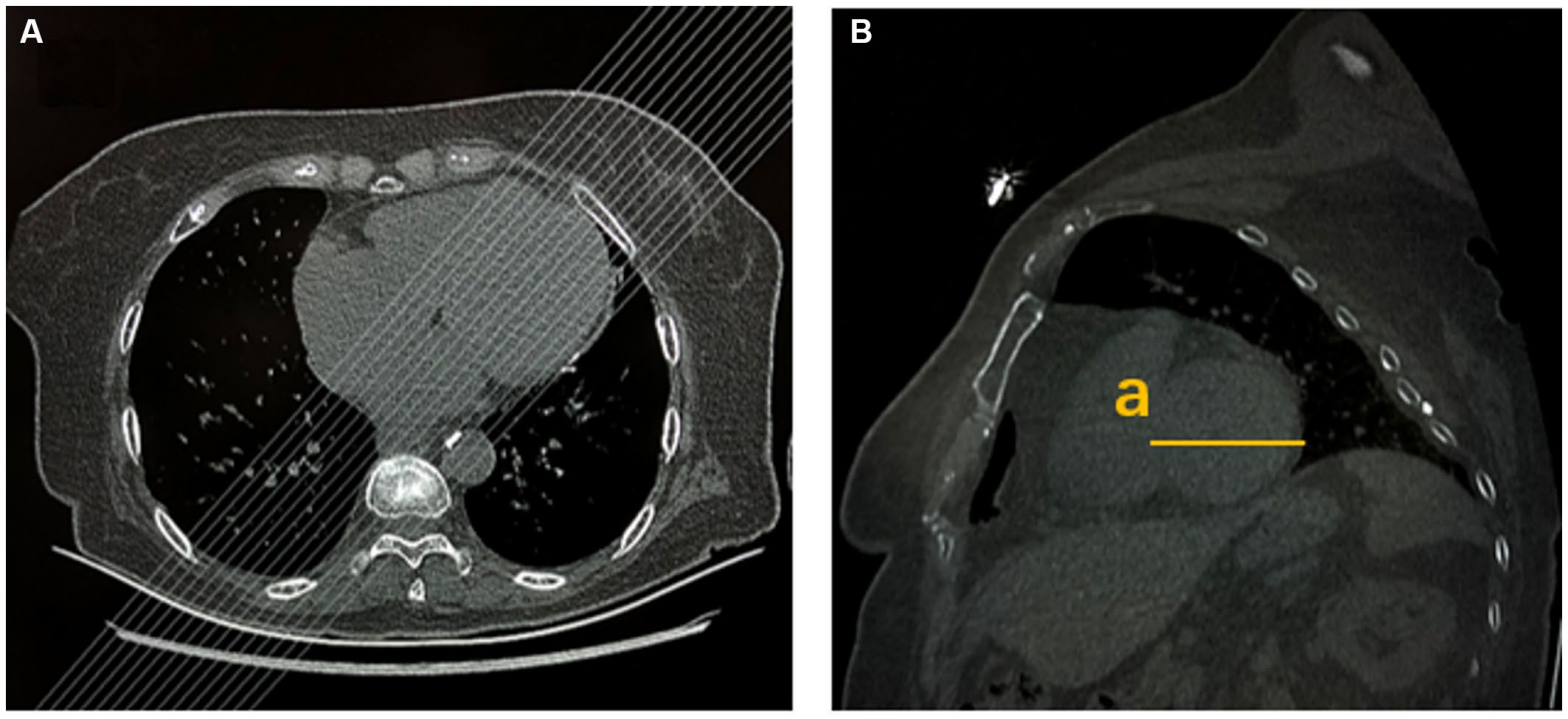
Left ventricular size was measured by chest CT. (A) Multiplanar reformation (MPR) after chest axial CT scans. (B) MPR after chest sagittal CT scans (a: maximum transverse diameter of left ventricular short axis, including interventricular septum and lateral wall).
